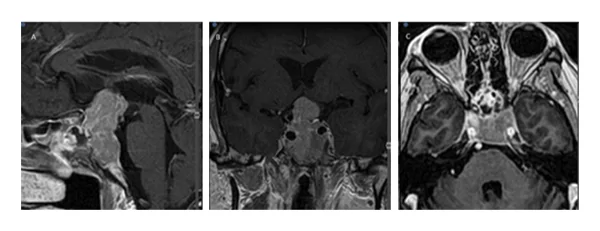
Preoperative MR images: (A–C) preoperative postcontrast T1‐weighted images demonstrate a recurrent pituitary adenoma with clival, sellar, suprasellar, and retrochiasmatic extension and compression of the optic chiasm. Axial (A), coronal (B), and sagittal (C) views show upward displacement of the third ventricle.
